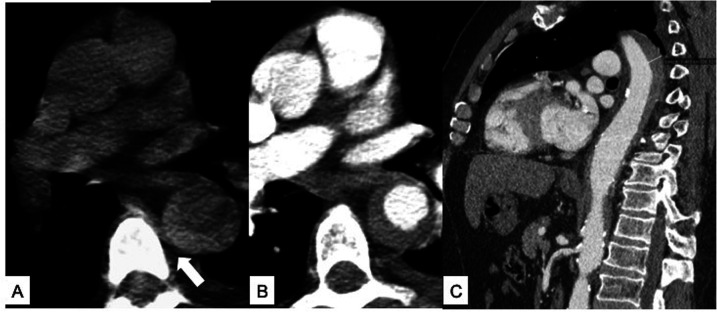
CT scan in a 62-year-old male patient with acute chest pain and Type B IMH. (A) Axial non-enhanced CT image shows hyperattenuating crescentic thickening of the aortic wall (arrow). (B) Axial contrast-enhanced CT scan shows a reduced diameter of the aortic lumen and the slick interface between the lumen and wall. (C) Contrast-enhanced sagittal multiplanar reformation CT image shows a reduced diameter of the aortic lumen and its constant circumferential relationship with the wall.
